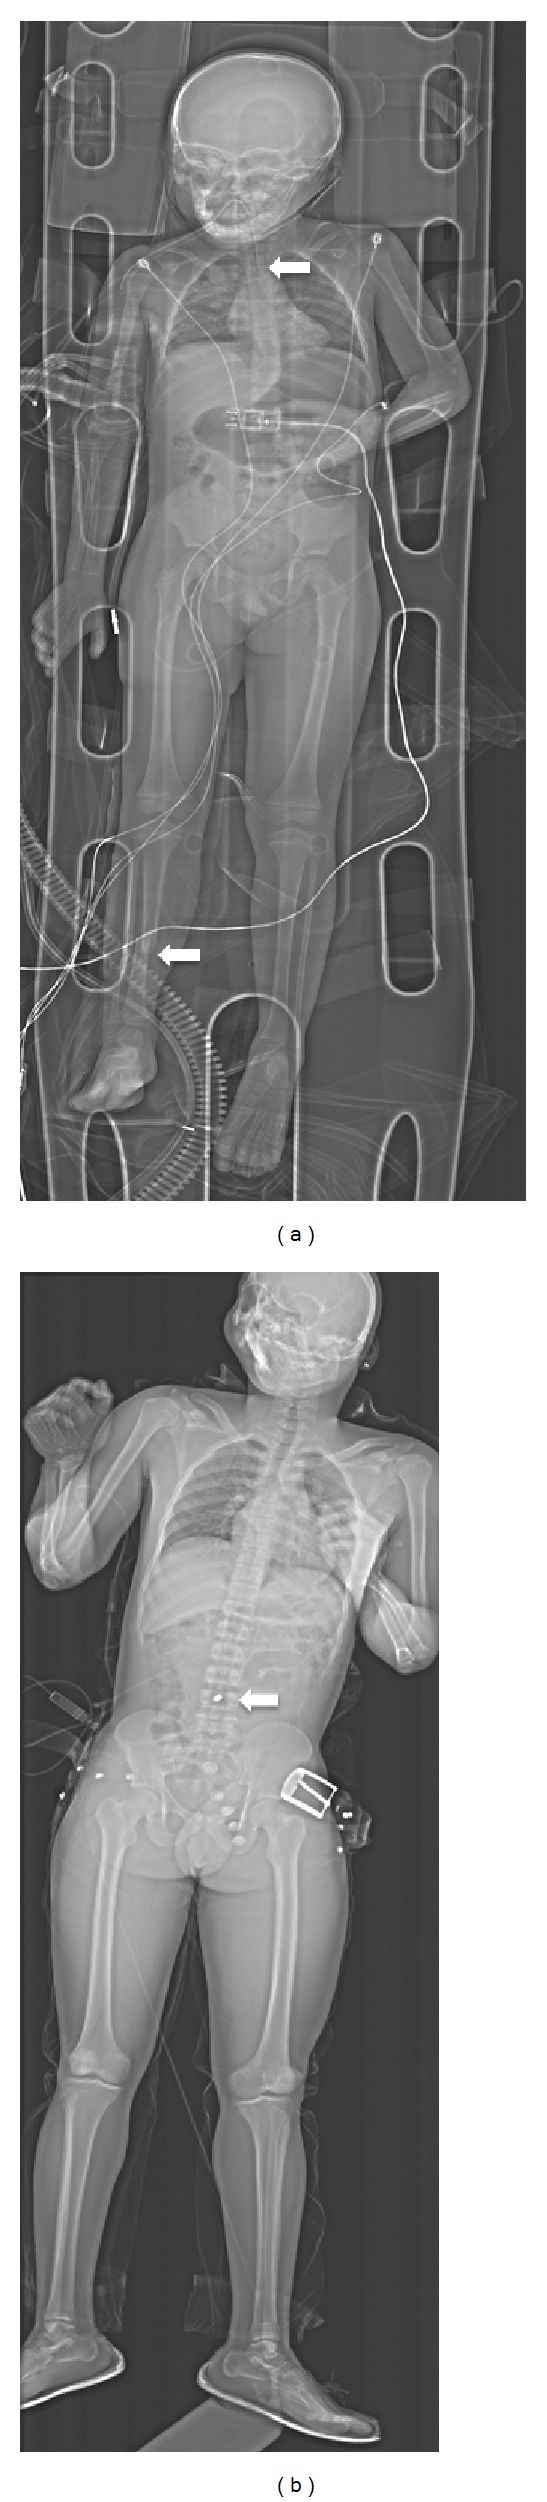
(a) LS X-ray of a paediatric patient showing fractured tibia/fibula and verification of chest intubation and (b) LS of an adult male showing gunshot wound to the abdomen.
